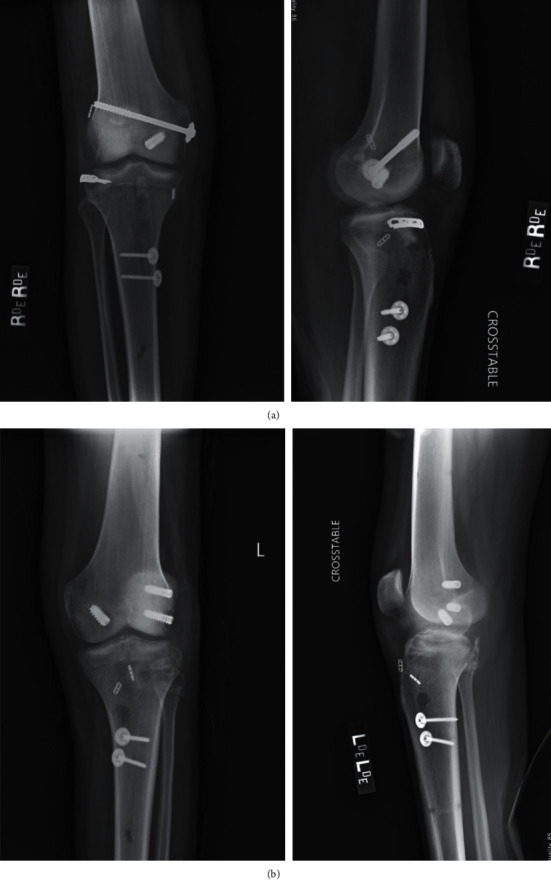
(a) Follow-up X-ray of the right knee. (b) Follow-up X-ray of the left knee.
